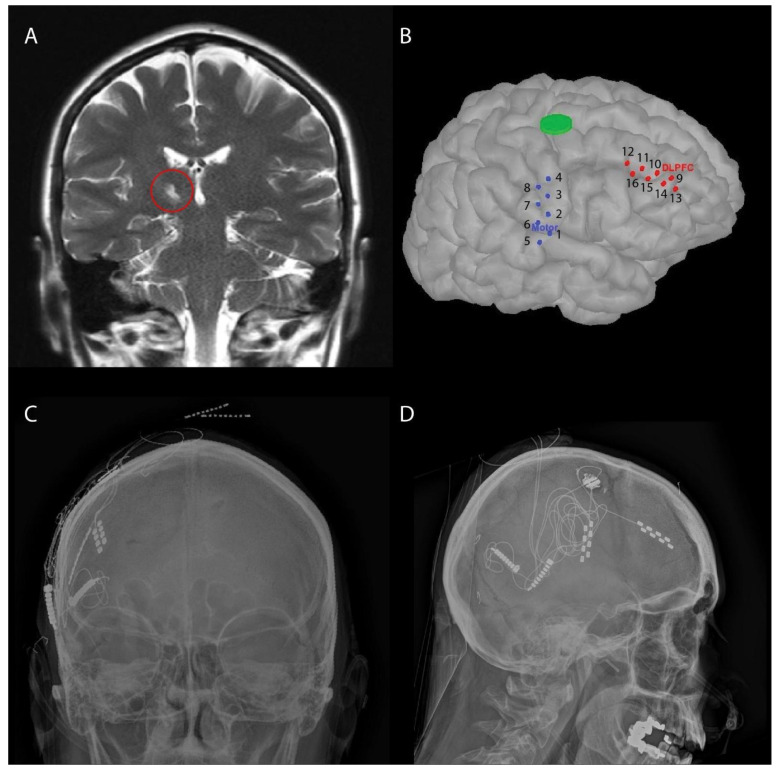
(A) T2 MR Coronal section of thalamic infarct (circled in red). (B) M1 and dlPFC electrode visualization by co-registration of the patient’s MRI and CT using SPM12 toolbox in Brainstorm [13,14], green circle is the burr hole location. (C) Anteroposterior X-ray projection of electrodes. (D) Lateral X-ray projection of the two Abbott Lamitrode 44 electrodes implanted on the patient’s right side. with the tunneled extensions seen superior and posteriorly.
